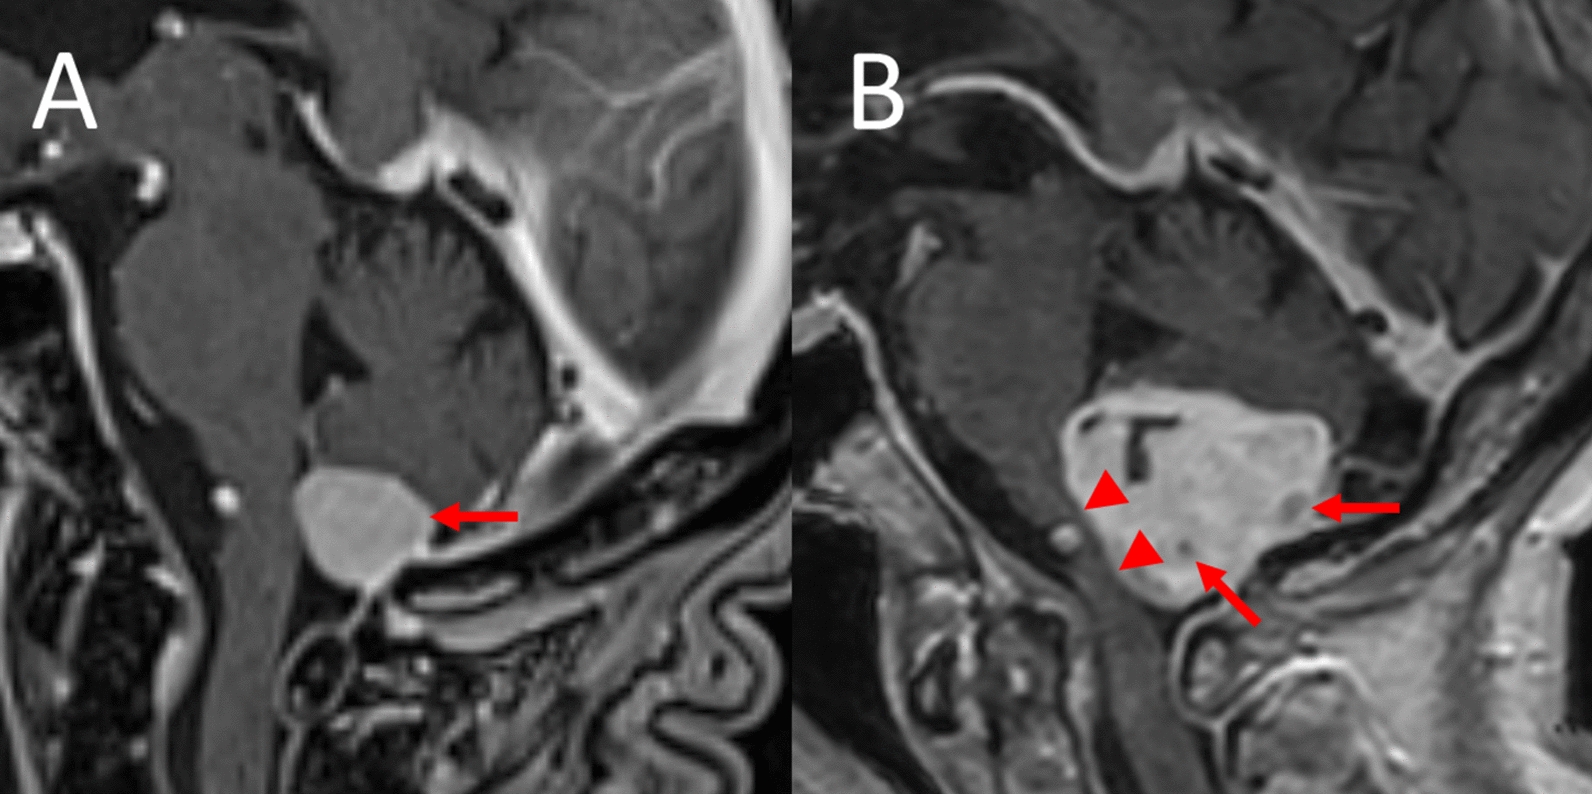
Growth of an infratentorial dorsal foramen magnum meningioma over 7 years (near median parasagittal T1-weighted contrast medium-enhanced magnetic resonance imaging sections). A Homogeneous avid tumoral contrast medium enhancement (arrow). B Tumor progression, inhomogeneous contrast medium enhancement with new areas of nonenhancement (arrows). Please note new subpontine brain stem compression on the follow-up scan (arrowheads)
